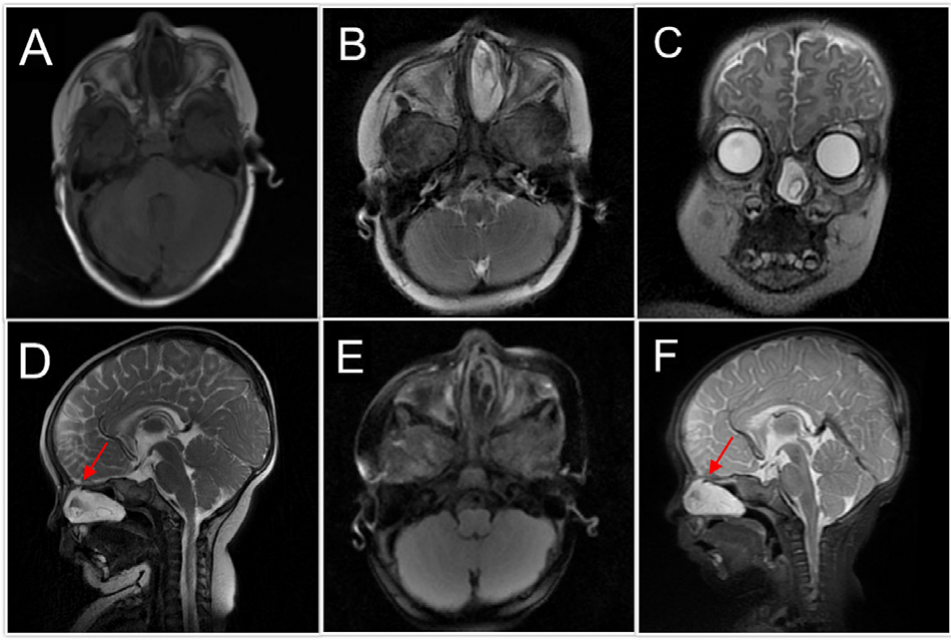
MRI imaging: (A) Axial T1, (B) Axial T2, (C) Coronal T2, (D) Sagittal T2, (E) Axial FLAIR, and (F) Sagittal STIR images show a left nasal cavity lesion with a low signal on T1 and high signals on T2 and STIR with loss of signal on FLAIR. A small hole in front of the skull base (foramen cecum) is indicated by arrows.
